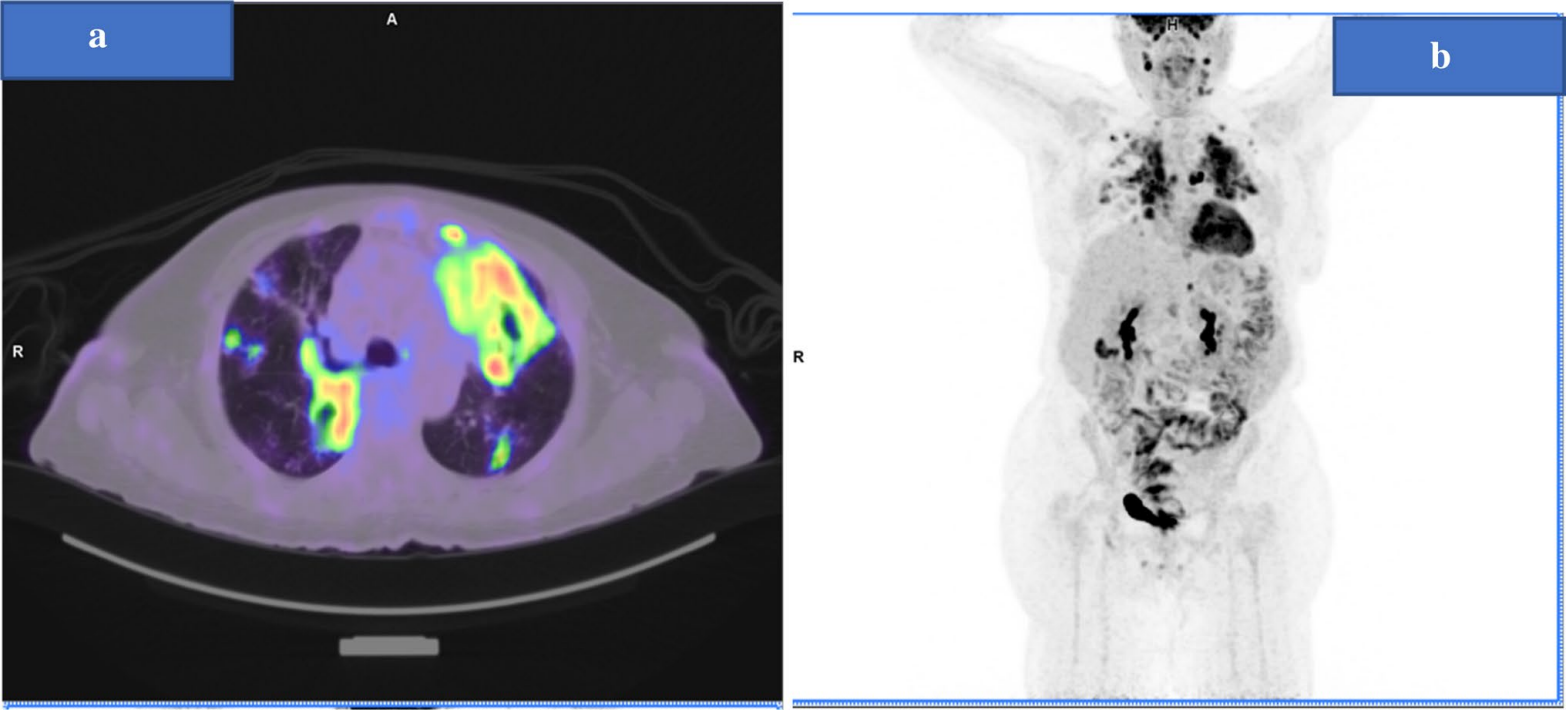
a, b PET CT (lung window) following six cycles of pembrolizumab showed mixed response with overall moderate progression
